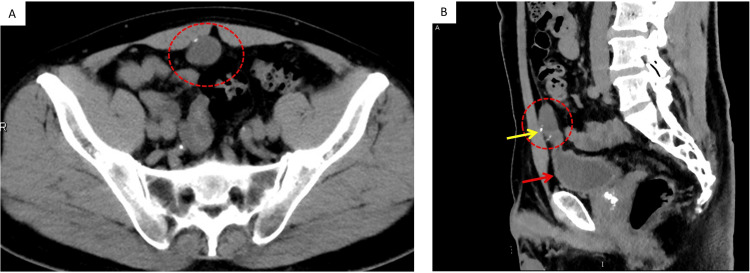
Computed tomography findings. (A) CT (axial plane): a low-density mass in the lower median abdomen behind the rectus abdominal muscle was observed (red dotted area); (B) CT (coronal plane): the mass (red dotted area) was located just cranial to the urinary bladder (red arrow) and seemed to connect to its top. Minimal calcification was observed (yellow arrow).
